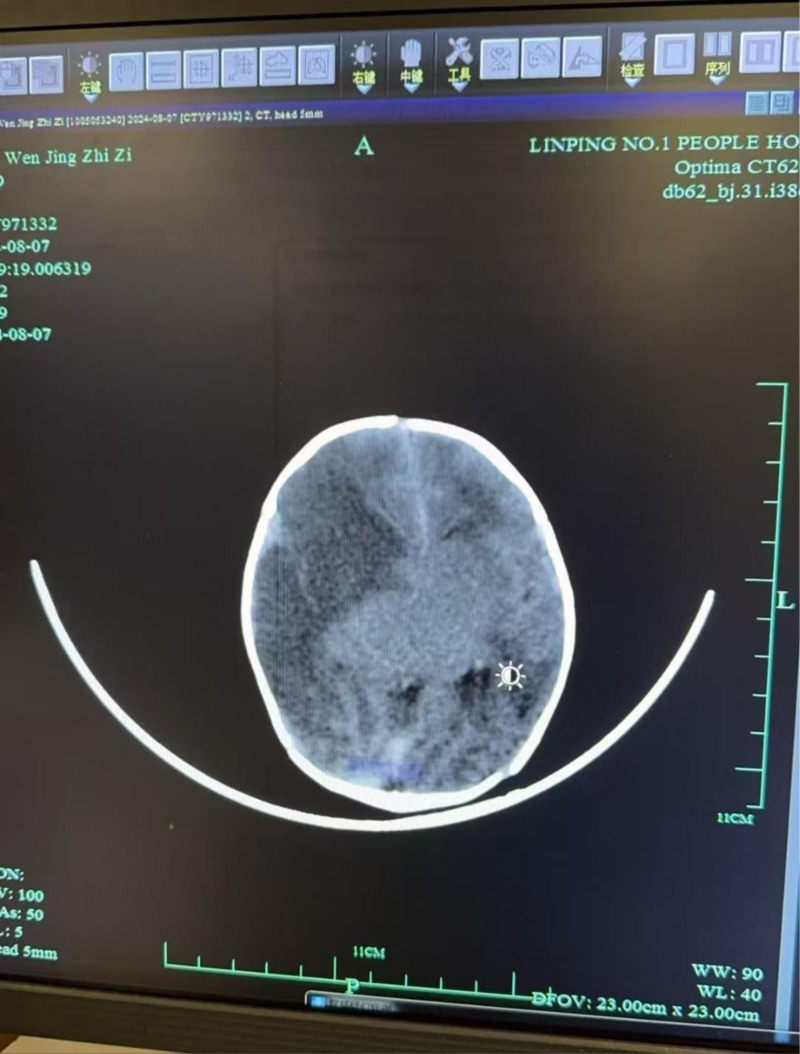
The CT of neonatal cerebral infarction. CT = computed tomography.
